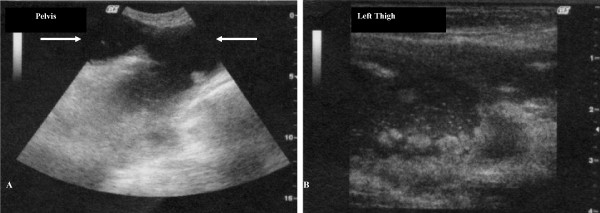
Transverse abdominal, pelvic and left thigh ultrasound. Hypoechoic collection with many internal echoes beginning on the left iliac fossa, involving the psoas muscle (arrows) (A) and extending inferiorly through the inguinal region until the root of the tight, with discrete infiltration of the subcutaneous tissues (B).
